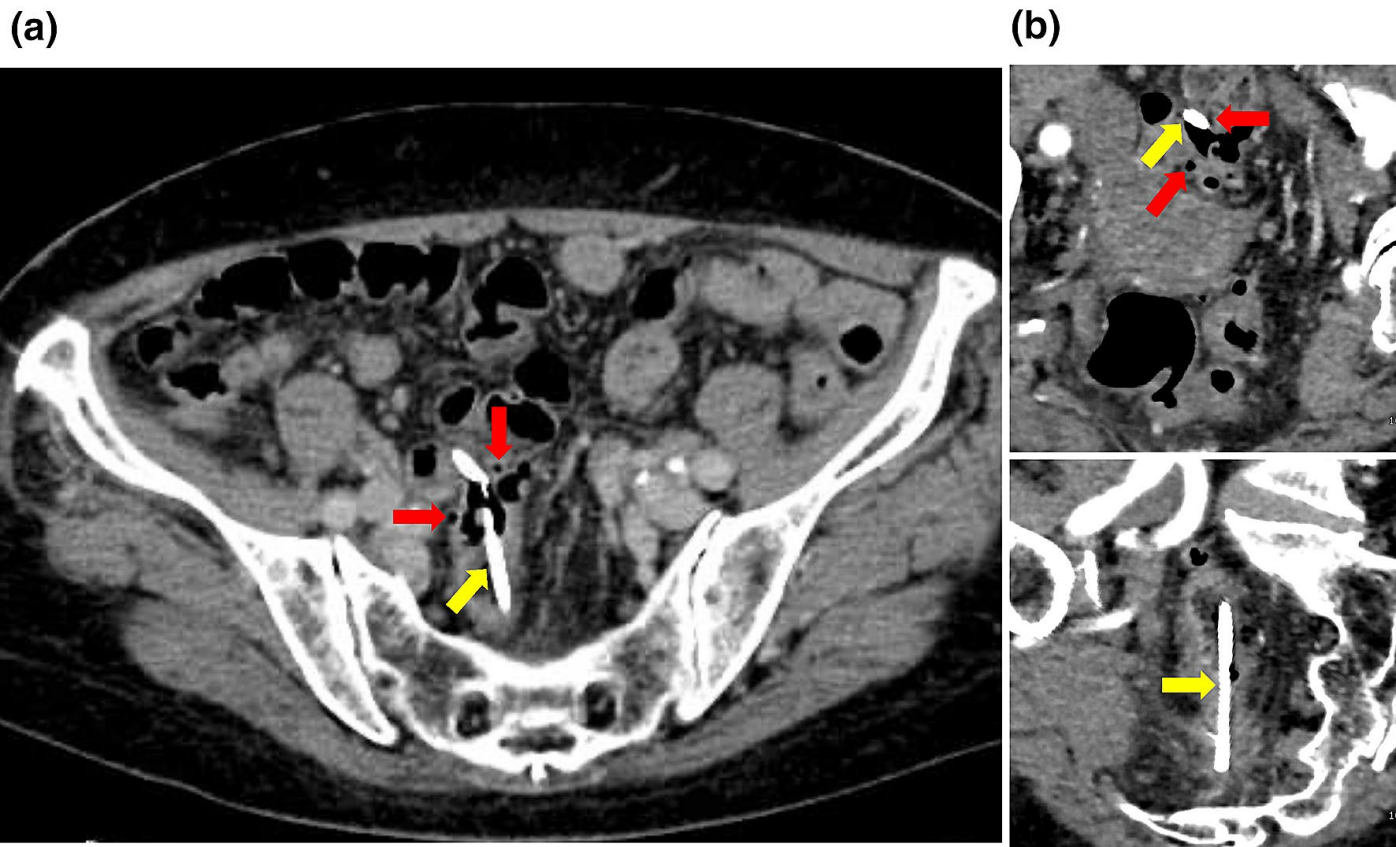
CT image of the abdomen on admission a Sigmoid colon perforation (yellow arrow) and the extraintestinal gas (red arrow) b Tip of the stent perforating the intestine (yellow arrow) and the extraintestinal gas (red arrow)
